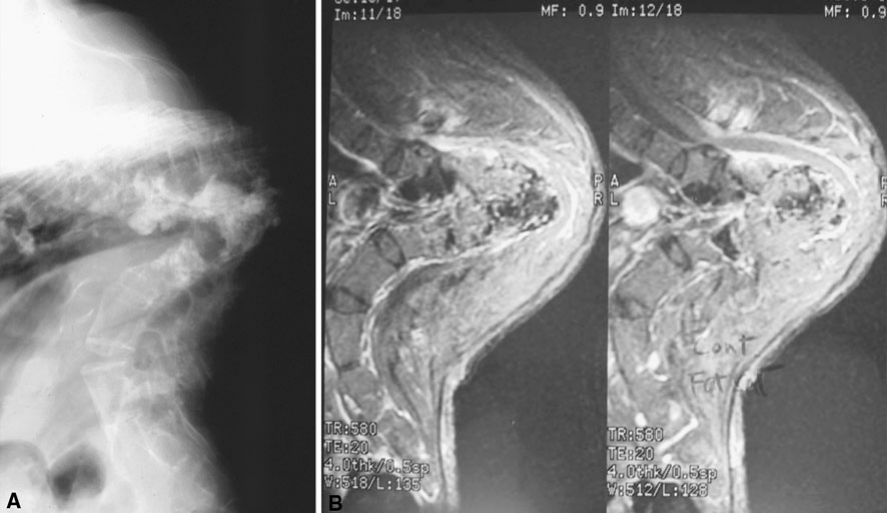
Late-onset Pott’s paraplegia with active disease. a Severe kyphosis over the thoracolumbar region with increased bone destruction. b MRI showed contrast enhancement at the kyphus
